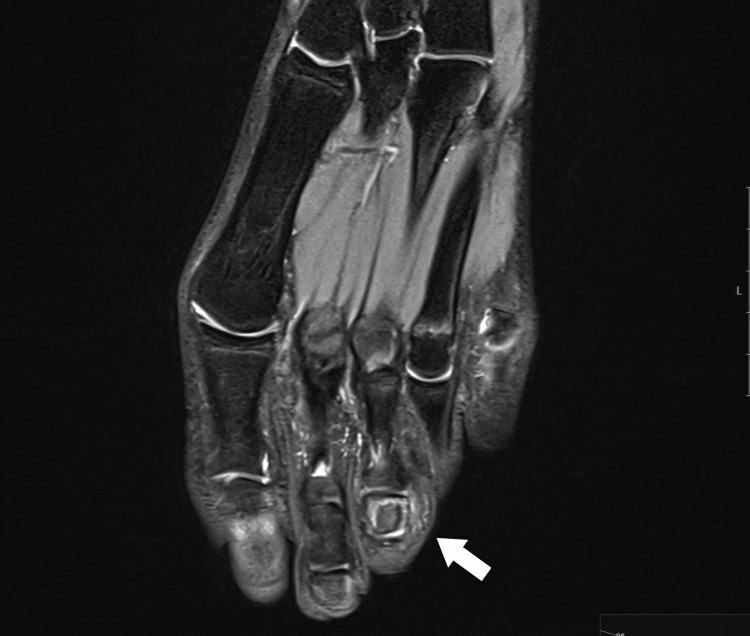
Axial T2-weighted MRI showing a centrally located high-intensity signal within the middle phalanx of the third toe, with a thin surrounding area of edema
